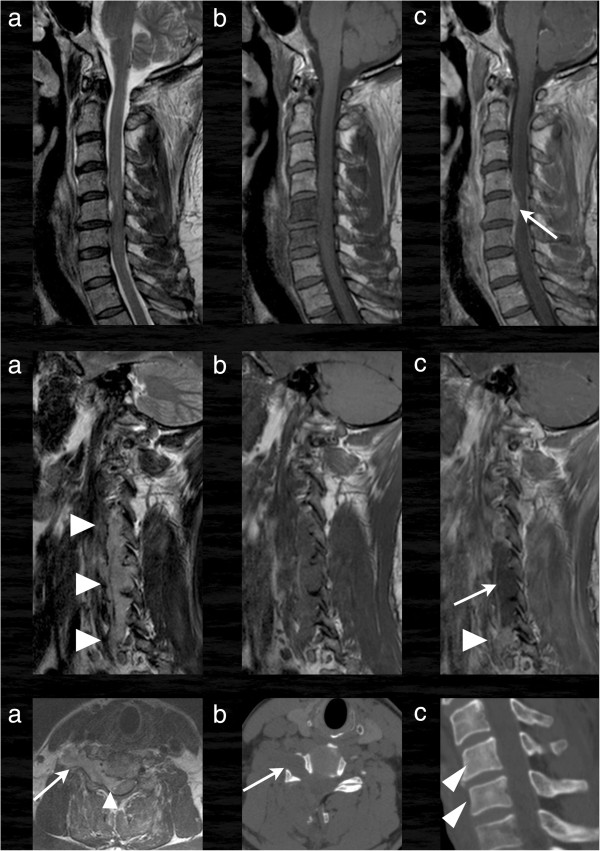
Cervical MRI and CT. In the first and second row sagittal and parasagittal images of the cervical spine in T2 (a), T1 (b) and T1 with contrast enhancement (c) are shown. There is a vertebral tumor infiltration of the fifth and sixth vertebra with a small invasion into the spinal canal (arrow). Larger tumor masses are found in the right lateral paravertebral space (second row). The tumor is hyperintens in T2 (a, arrowheads), hypointens in T1 and is partially contrast enhancing (arrowhead) with central non-enhancing parts (arrow in c). Remarkably the tumor grows through the intervertebral foramina in an hour glass pattern (last row, a) like a schwannoma even with enlargement of the osseous canal (arrow in b, axial CT). The infiltrated vertebrae are only slightly hyperdens compared to the normal vertebrae (arrowheads in c).
